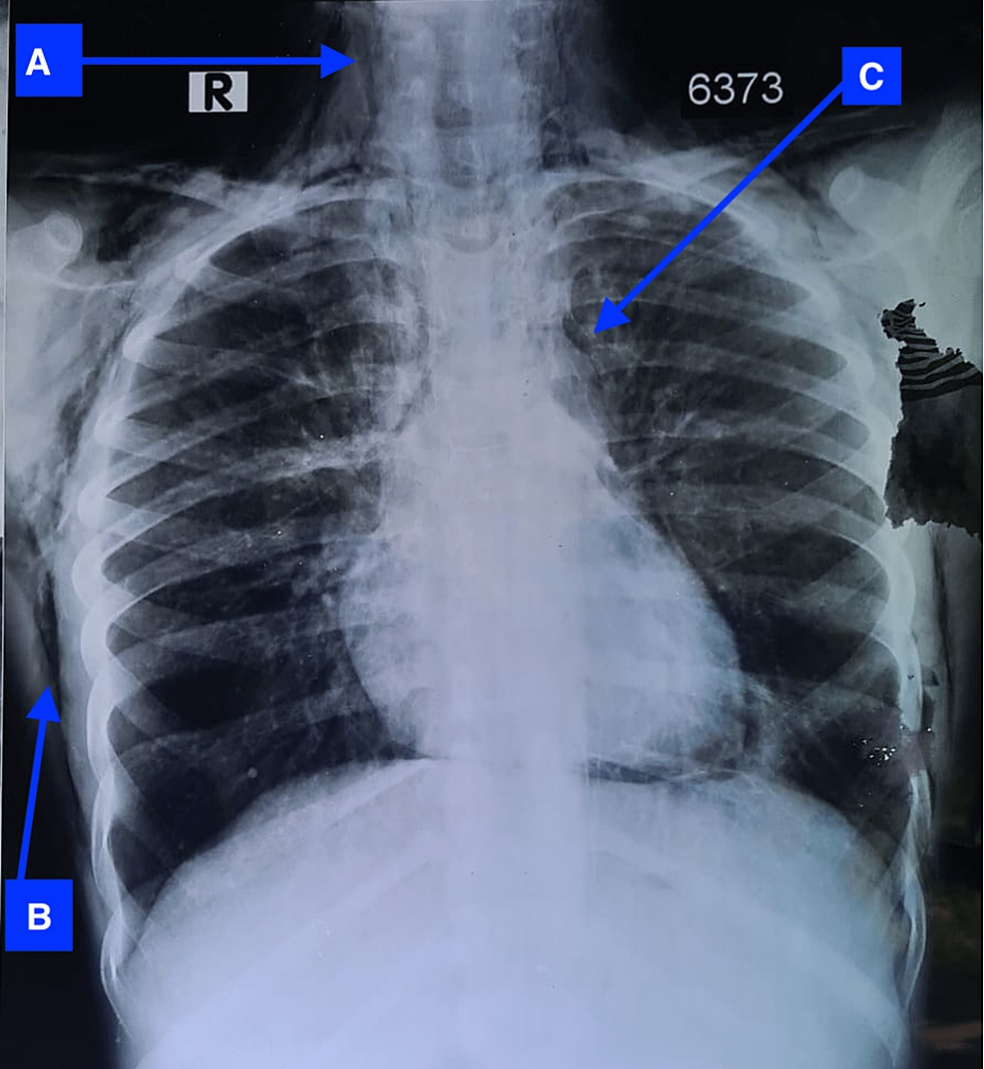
Chest radiograph at the time of initial presentation There is air in neck (A), subcutaneous tissues (B) and mediastinum (C)
